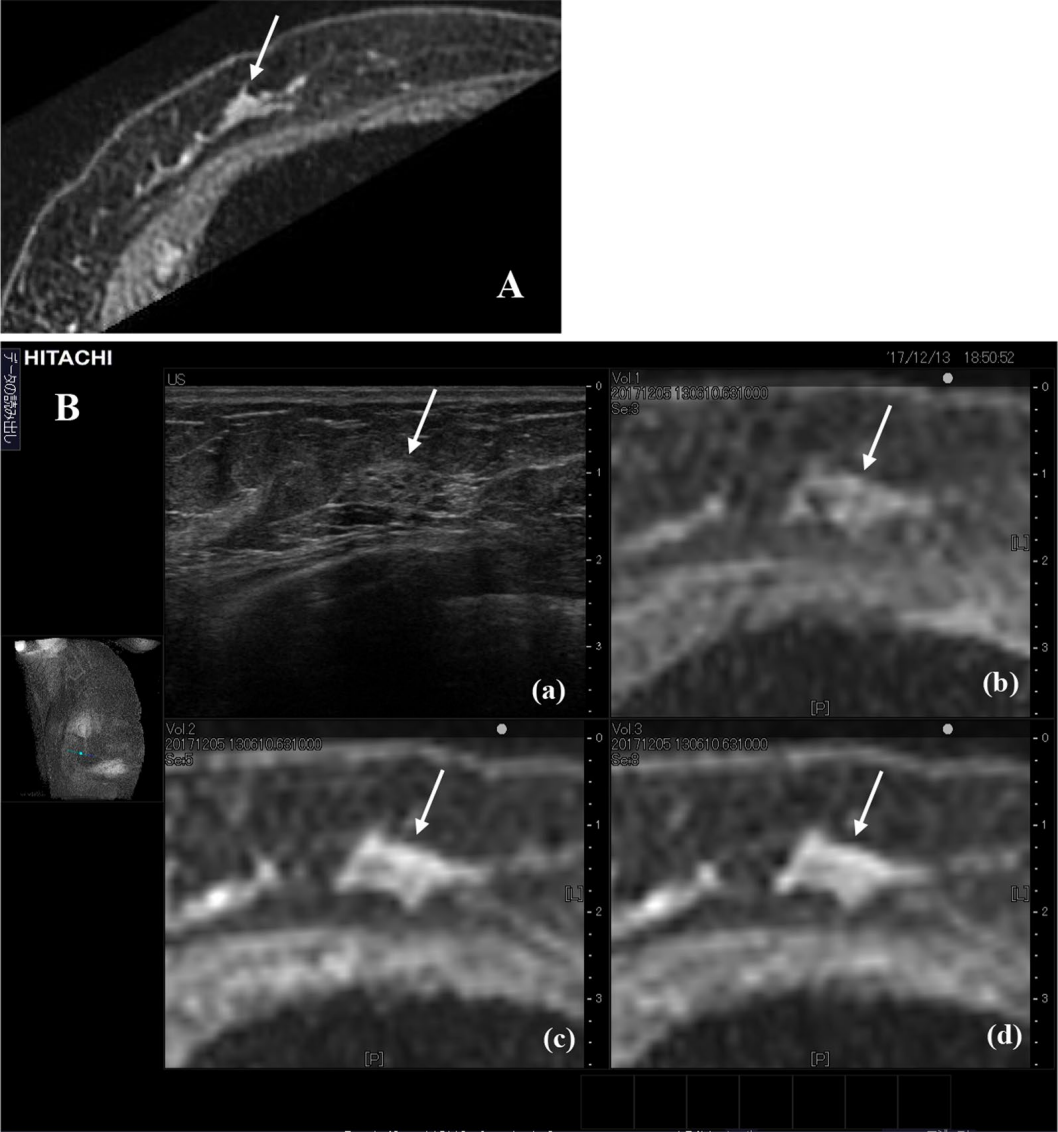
A T1-weighted, contrast-enhanced additional supine MRI with multi planar reconstruction (MRI-MPR) showed an irregular enhancing mass (arrow). B View from the RVS monitor. Sonography showed a heterogeneous mass with low echogenicity and irregular shape. A pre-contrast MRI-MPR image is shown in (b). An early phase MRI-MPR image is shown in c. A late-phase MRI-MPR image is shown in d. Images (b–d) correspond to sonographic cutaway images in a
